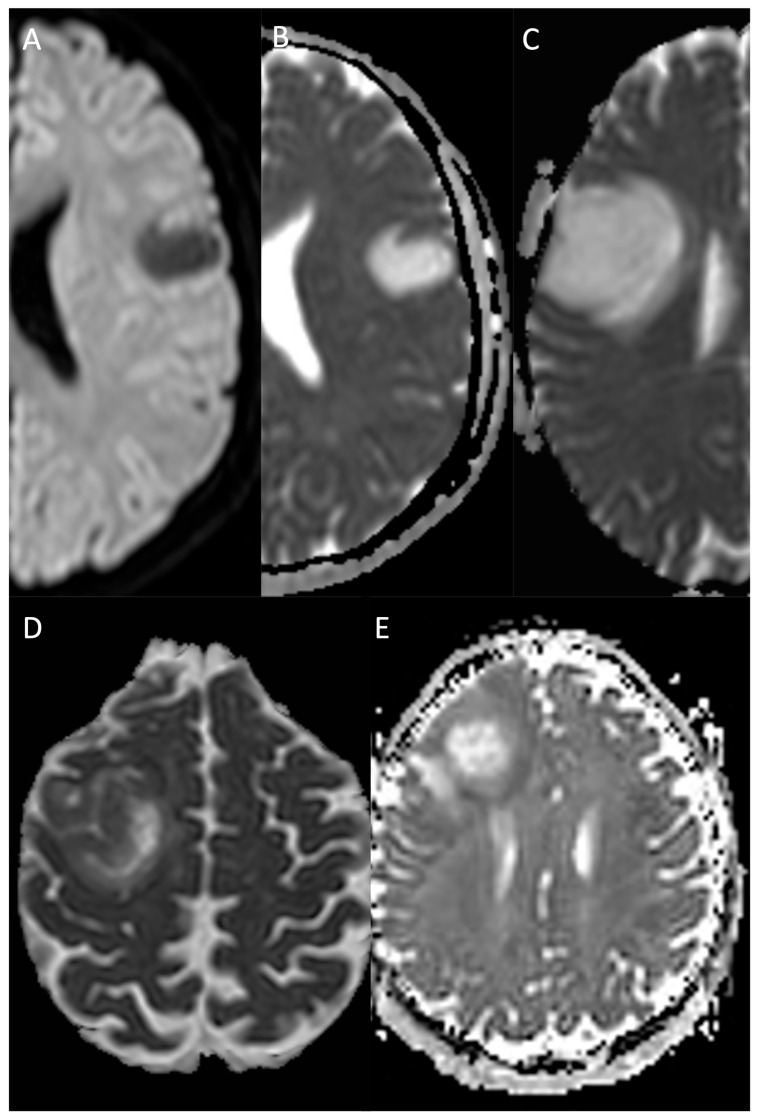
Compares the DWI/ADC sequences of patients affected by an incidental grade 2, IDH-mutant diffuse astrocytoma involving the inferior and lateral (fronto-opercular) portion of the left frontal lobe (A,B), a grade 2, IDH-mutant diffuse astrocytoma involving the fronto-opercular portion of the right frontal lobe (C), a grade 4, IDH wild-type primary glioblastoma of the premotor area of the right frontal lobe (D), and a grade 4, IDH wild-type primary glioblastoma of the right frontal lobe (E). LGGs (A–C) do not show diffusion restriction, while HGGs (D,E) demonstrate significant diffusion restriction of the peripheral vital areas. *DWI: diffusion-weighted image; ADC: apparent diffusion coefficient; LGG: low-grade glioma; HGG: high-grade glioma*.
